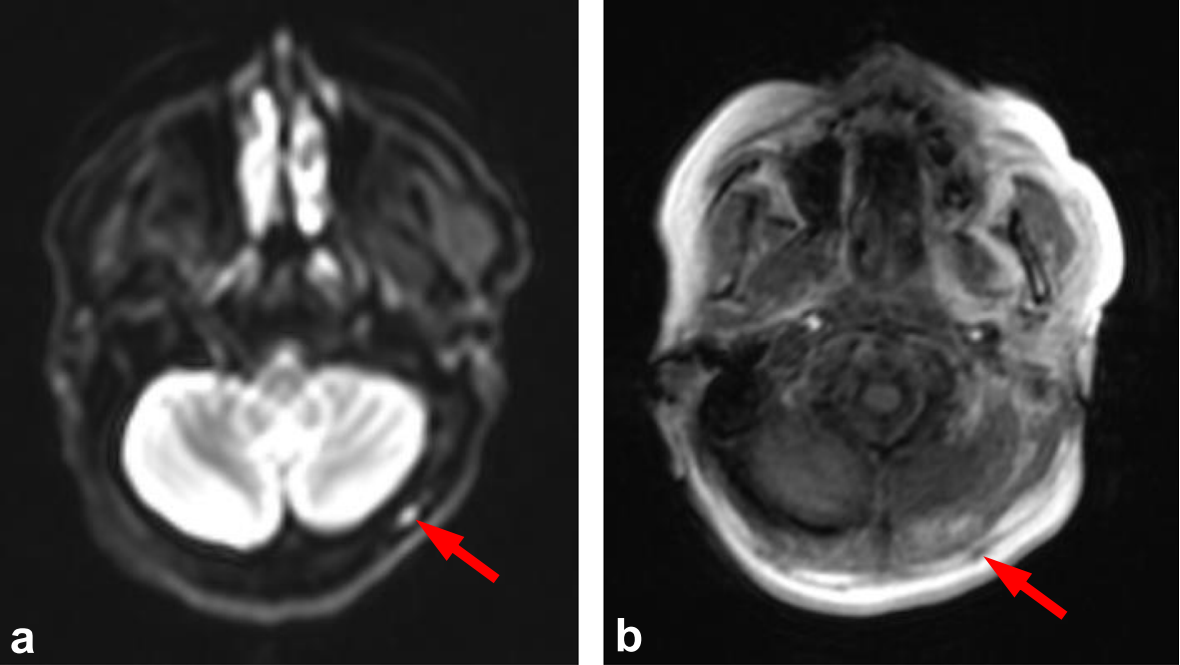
Thickening of subcutaneous facia. Observer score variation within the skull due to thickening of subcutaneous fascia (arrows) shown by (a) axial b900 DW MRI and (b) axial in-phase Dixon MRI. High diffusion signal leads to a false positive report of a skull lesion (arrow a), in-phase Dixon images show the lesion to not be within the skull and instead relate to thickening of subcutaneous facia (arrow b). Synonymous to this we also found sub-occipital lymph nodes can lead to false positive reporting of focal lesions within the skull
